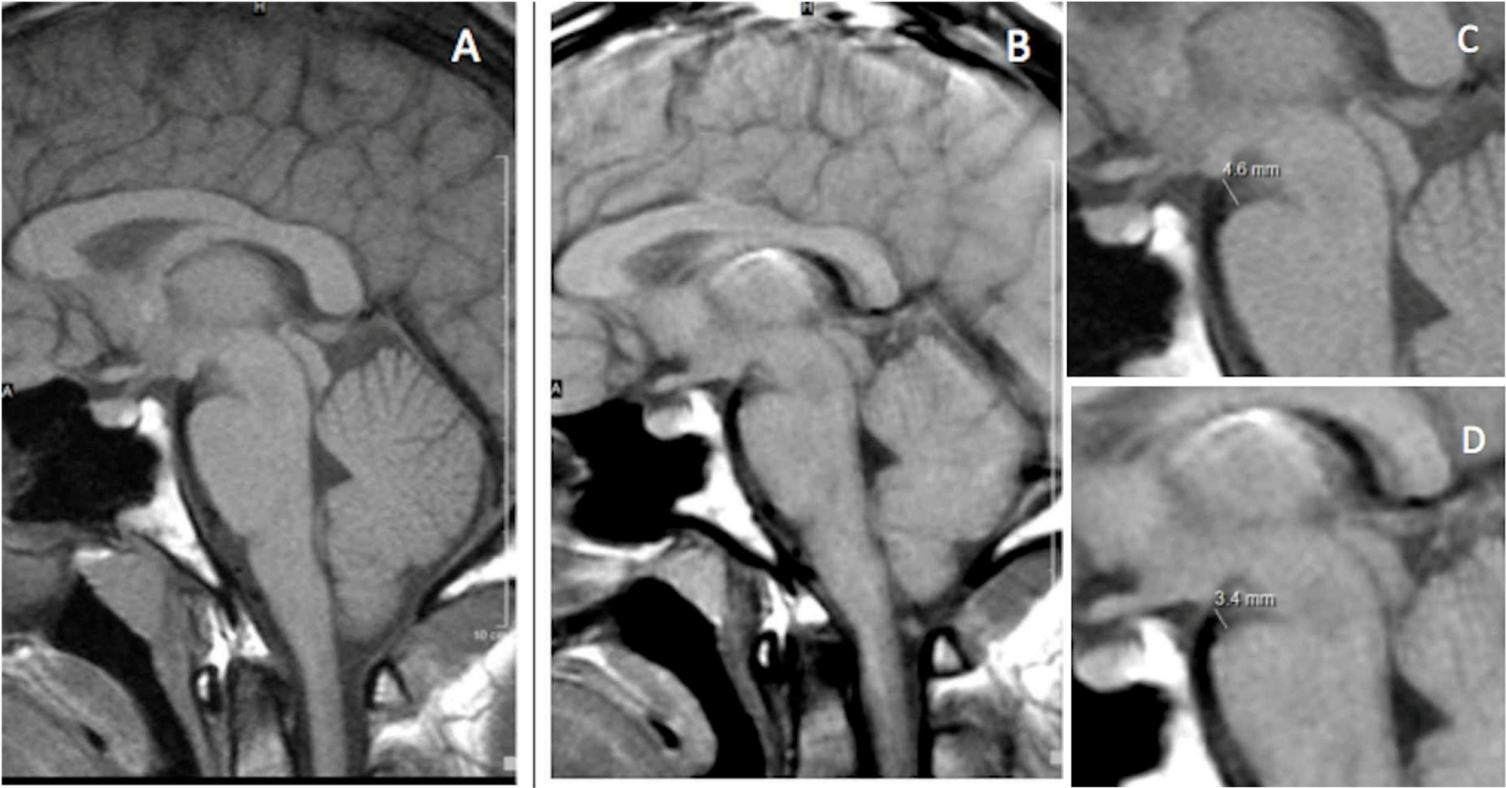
Case 2 MRI progression (A) MRI brain obtained from a concussion that had occurred 13 months prior to current injury, with a measured mamillopontine distance of 4.6 millimeters (highlighted in image C). (B) MRI brain obtained from current concussion showing a decreased mamillopontine distance of 3.4 millimeters (highlighted in image D). The observed decrease of the mamillopontine distance is a finding in intracranial hypotension.
